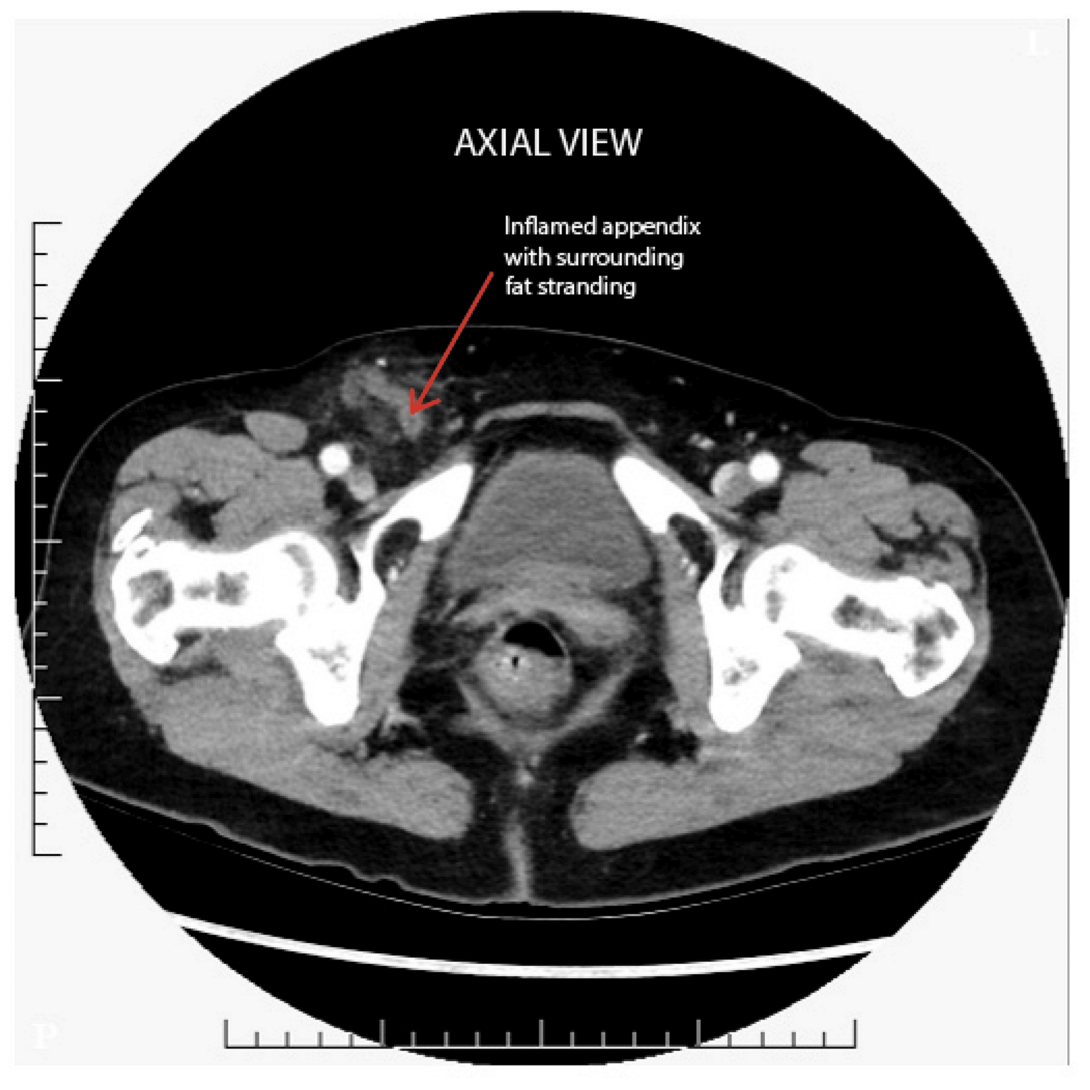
CT axial view of De Garengeot hernia with inflamed appendix and surrounding fat stranding
